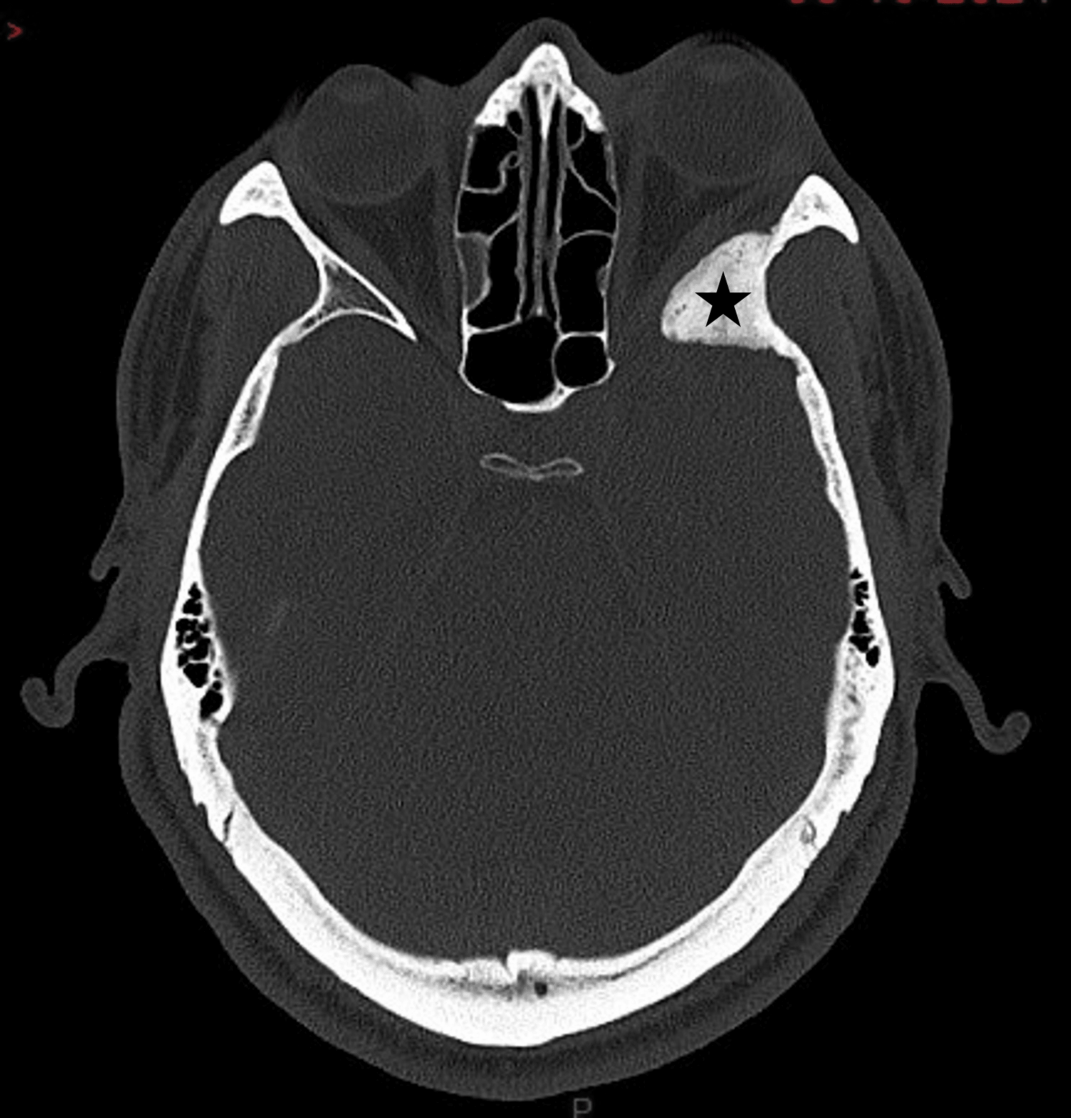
Preoperative axial non-contrast brain CT in bone window. Thickening and hyperostosis of the left greater wing of the sphenoid (black star). The margins of the hyperostosis are smooth.
